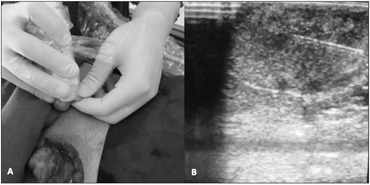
Tumor marking (A) for intraoperative ultrasound examination and needle driving (B).
